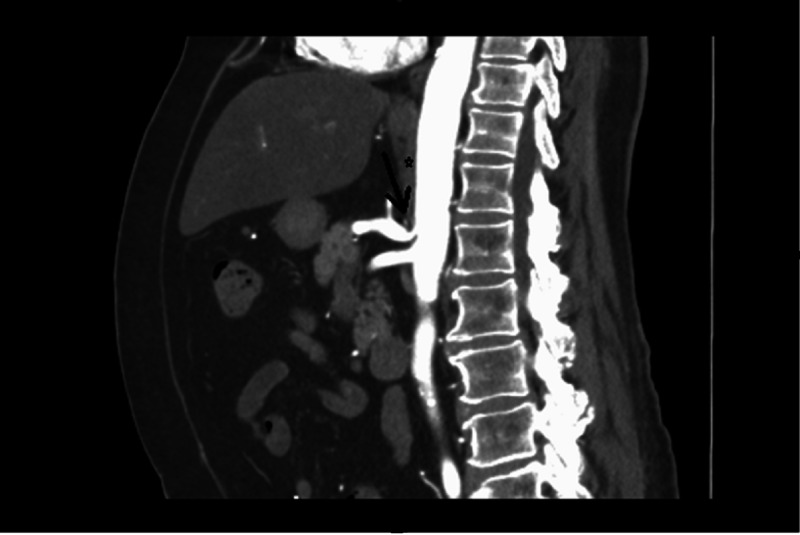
Sagittal CT angiogram of the abdomen. The image shows that median arcuate ligament (star) is located anterior to the celiac trunk and the classic compression can be seen proximal to the celiac trunk with post stenotic dilatation (black arrow).
